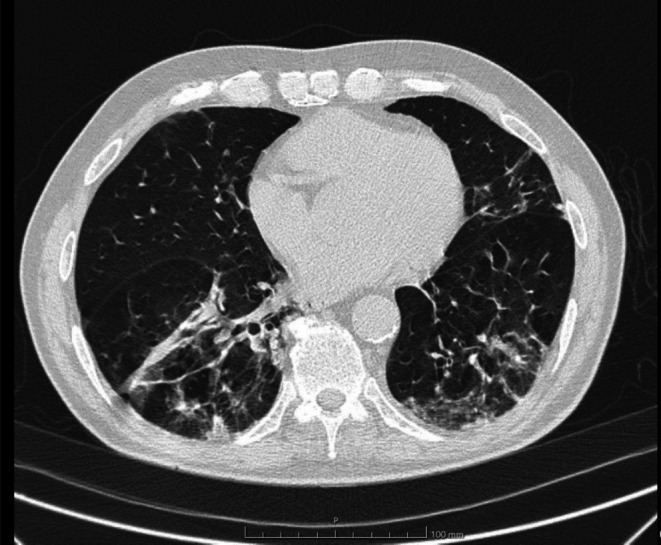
CT scan—extensive areas of consolidation, with peribronchial distribution and subpleural OOP‐like aspects, which are associated with post‐obstructive atelectatic aspects in the apical segment of the LIL, fuzzy “tree‐in‐bud” centrilobular micronodularities, and bronchial wall thickening.
